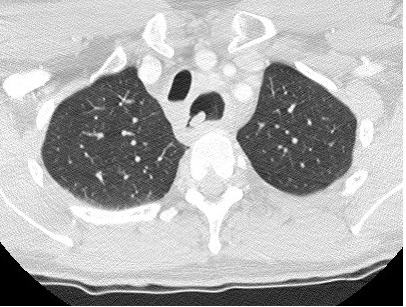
Oesophageal thickening and intraluminal mass seen on computed tomograghy (lung windows).
